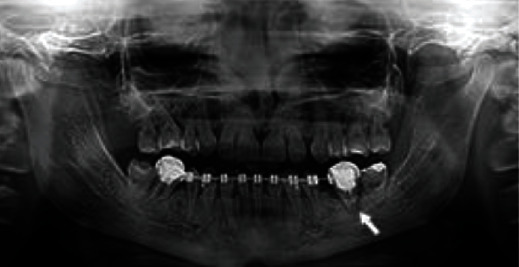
Panoramic radiograph taken at age of 29 right before prosthodontic treatment.
